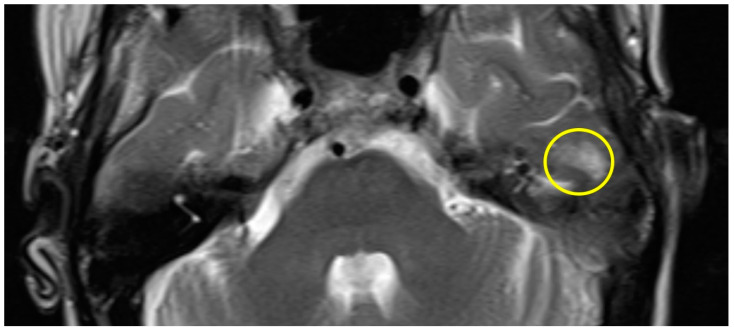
Axial T2w MR imaging of patient #1 (yellow circle = gliosis). Supplementary MR imaging can be downloaded.
